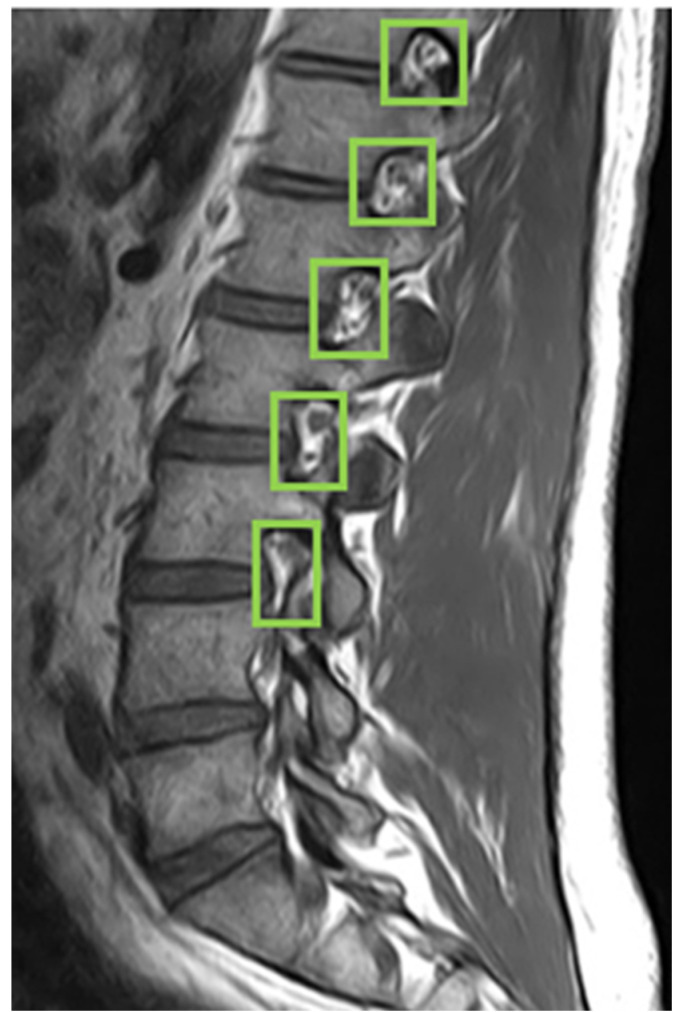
Use LabelImg tool to annotate intervertebral foramen MRI image as green boxes.
